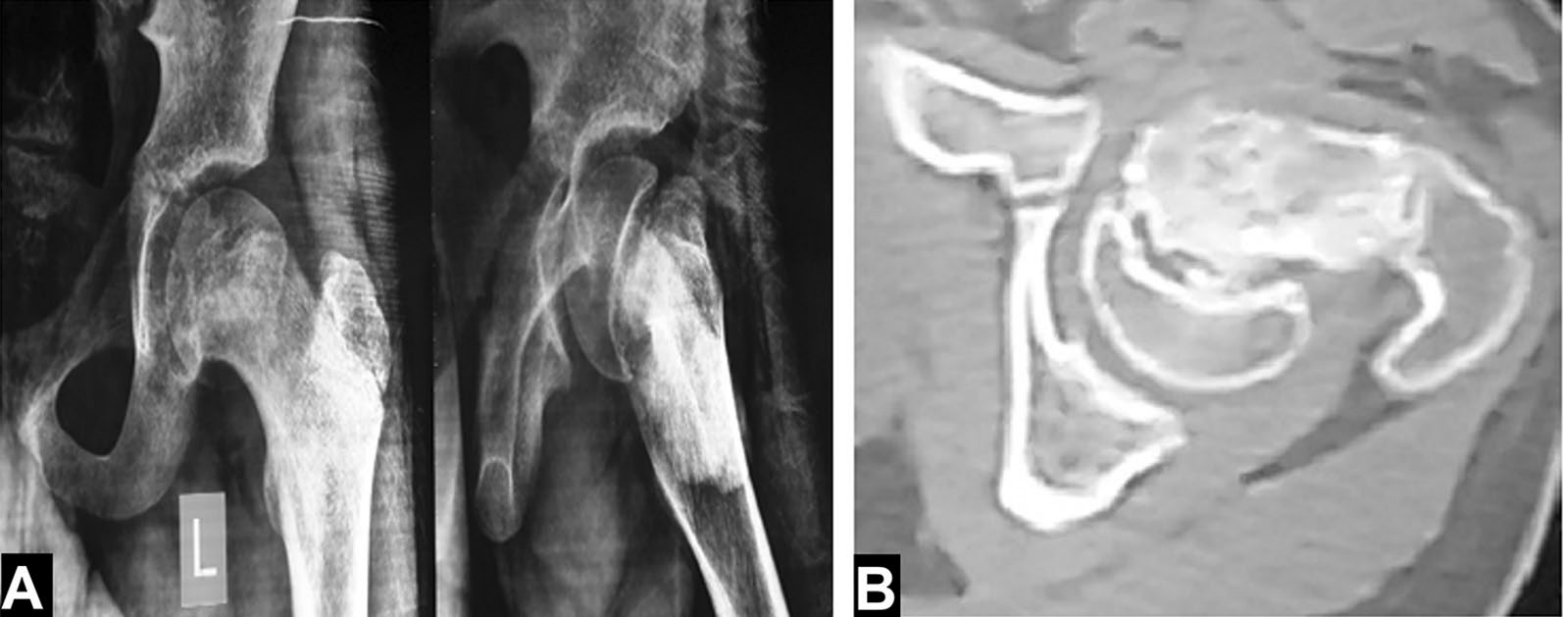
Preoperative images of a 13-year-old male patient with left chronic SCFE. A Plain X-rays (AP and lateral views). B CT axial cut
